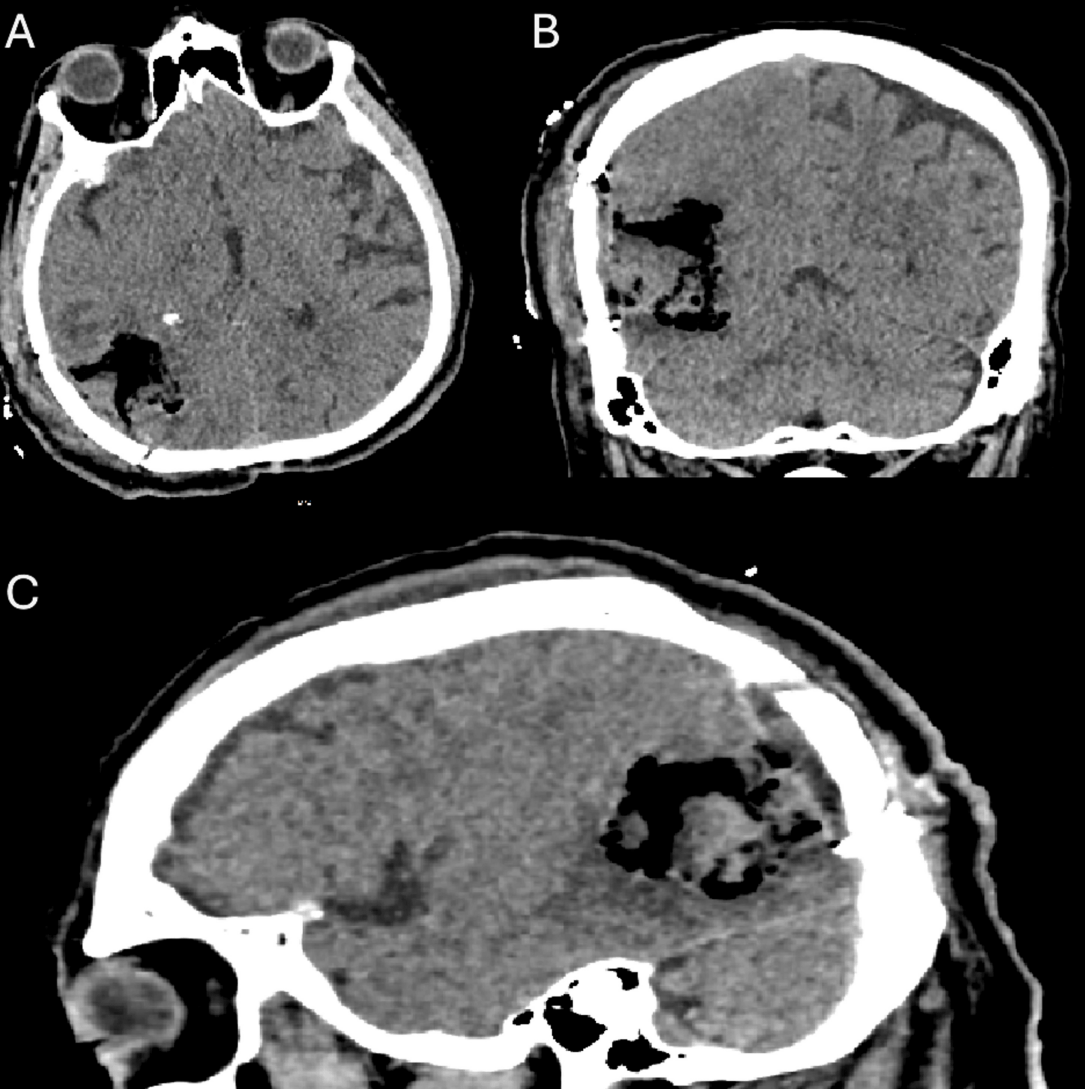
Post-operative CT scan The post-operative CT scan demonstrates the tumor cavity in three planes: axial (A), coronal (B), and sagittal (C).
